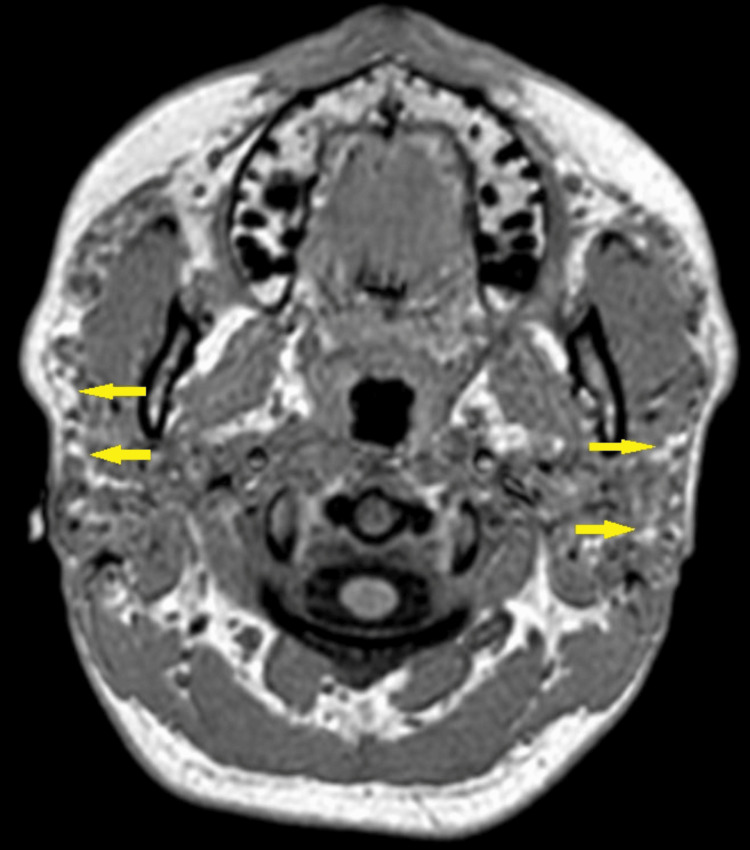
T1-weighted image depicts grid-like fat signals (yellow arrows), best seen on the right parotid gland. Staging system for conventional imaging of the parotid glands proposed by Rao et al. [14], grade 2.
